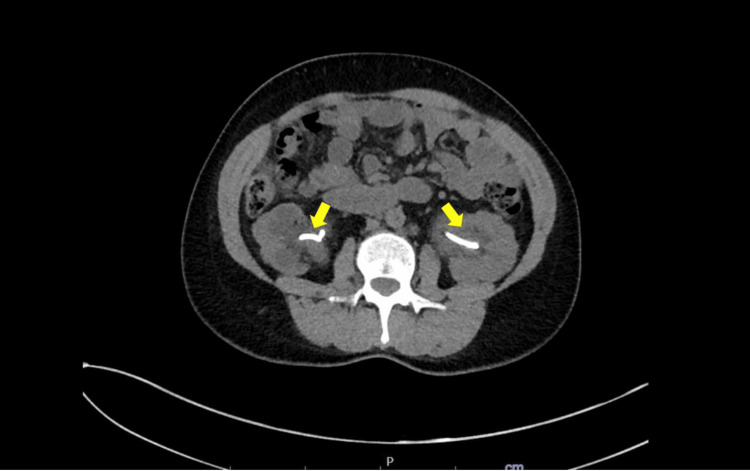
Cross-sectional view of CT abdomen and pelvis showing the resolution of obstruction after placement of ureteral stents.
